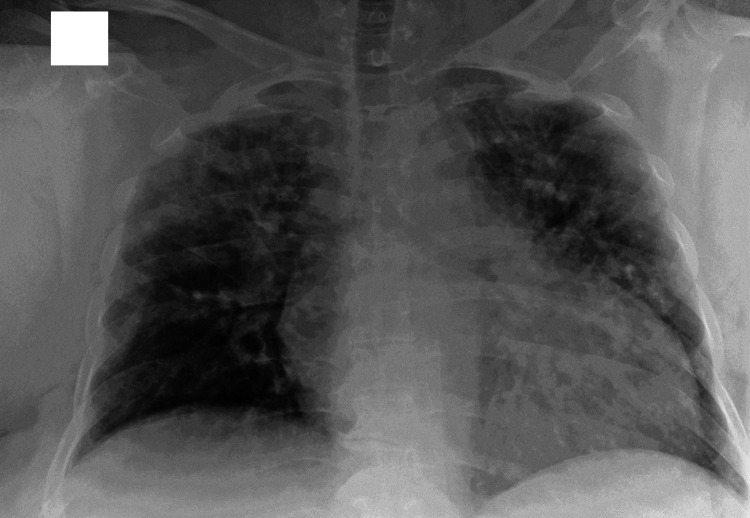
The radiographic examination demonstrates a widespread increase in the peribroncovascular interstitial texture with associated multiple parenchymal thickening areas arranged in correspondence with the upper field of both lungs. Heart increased in volume; sinus costophrenics hypo-expanded but free from effusion. The x-ray picture confirms the presence of bilateral interstitial pneumonia strongly suspected for a positivity to COVID-19.
